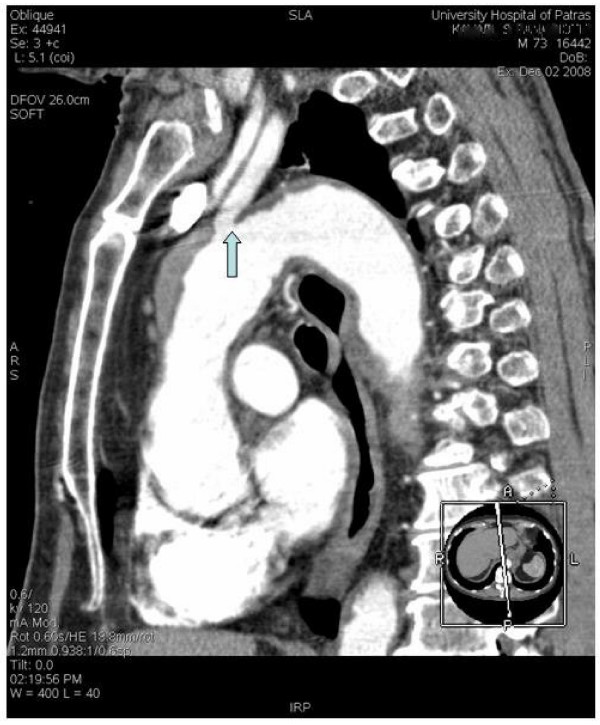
Oblique reformation image of a contrast-enhanced CT scan of the same patient. The IMH is appeared as a thickening of the aortic wall extended in the aortic arch compressing the origin of the brachiocephalic artery (arrow).
